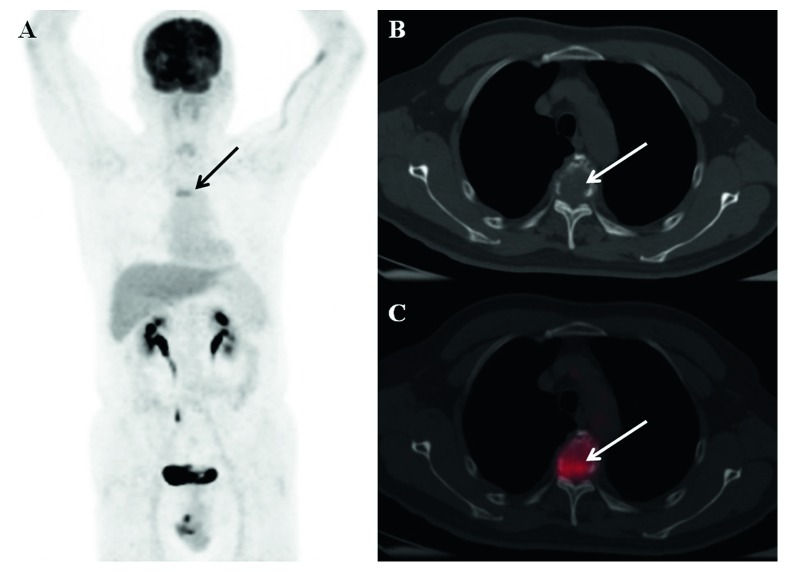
^18^F-FDG PET/CT imaging in a 60-year-old man with MM: (a) MIP image; (b-c) transaxial CT and fused images. In this patient, the descriptive criteria (IMPeTUs) were BM(2), F2.SP(4), L2. ^18^F-FDG PET/CT showed increased ^18^F-FDG uptake in the T4 spine (arrows). His disease stage was IA by DS Plus. The serum *β*_2_-microglobulin level was 6.08 mg/L, and the LDH level was 310 U/L. The patient was classified as III according to the RISS.
